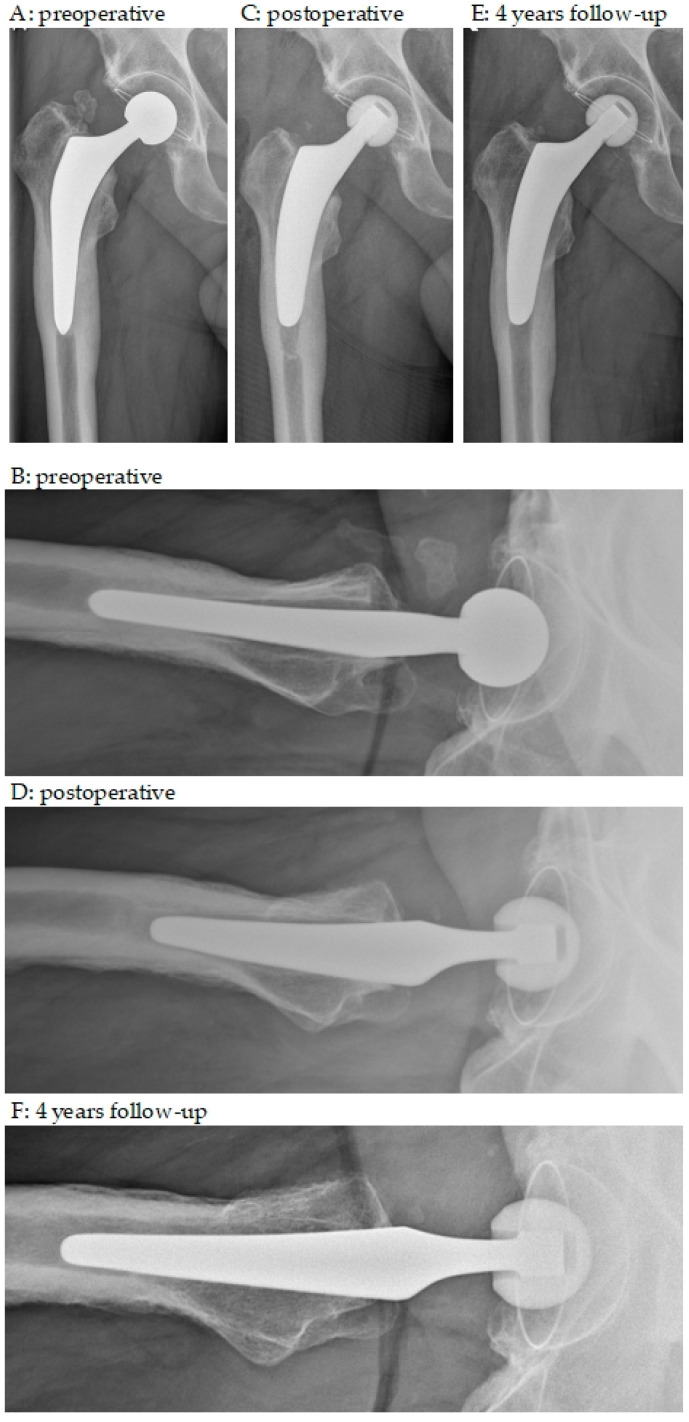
Anteroposterior and axial X-rays. (A,B) preoperative; (C,D) postoperative; (E,F) 4 years follow-up. A 66-year-old male with increasing pain and immobilization 5 years after THA; progressive stress shielding distally around the stem and loosening zone according to Gruen I, II and VI, VII as well as VIII, IX and XIII, XIV; no infection parameters and negative joint aspiration; stem revision with cementless short stem; in particular, in the axial view, the broader geometry of the short stem compared to the explanted stem allows sufficient bone stock and provides adequate rotational stability.
